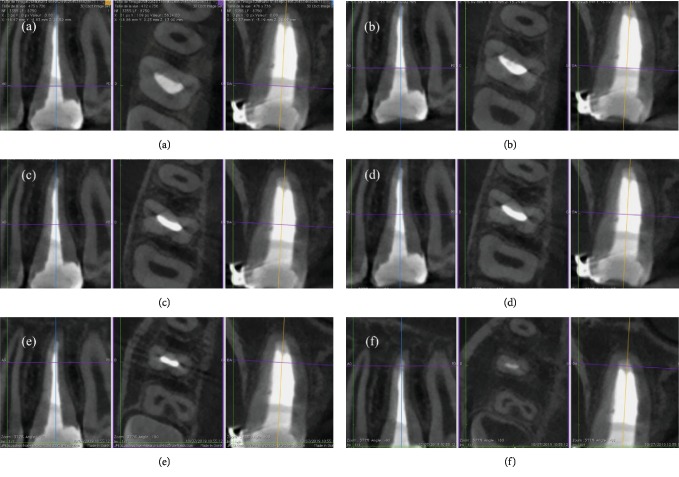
(a–f) CT images of the tooth at different level display, without any doubt, the C1 configuration of this maxillary right molar.
